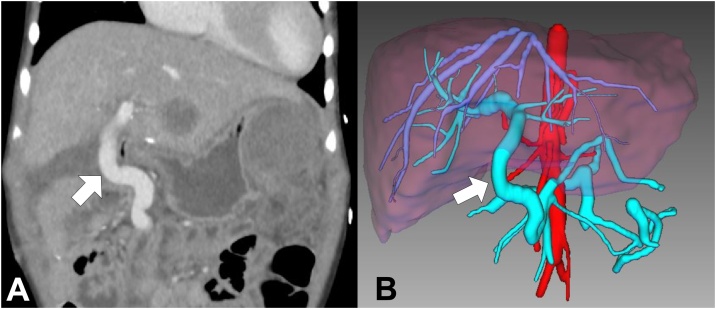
A) CT scan 1 month after the re-do MRB showing a patent conduit (arrow). B) 3D reconstruction of the re-do MRB 1 month after the operation.
